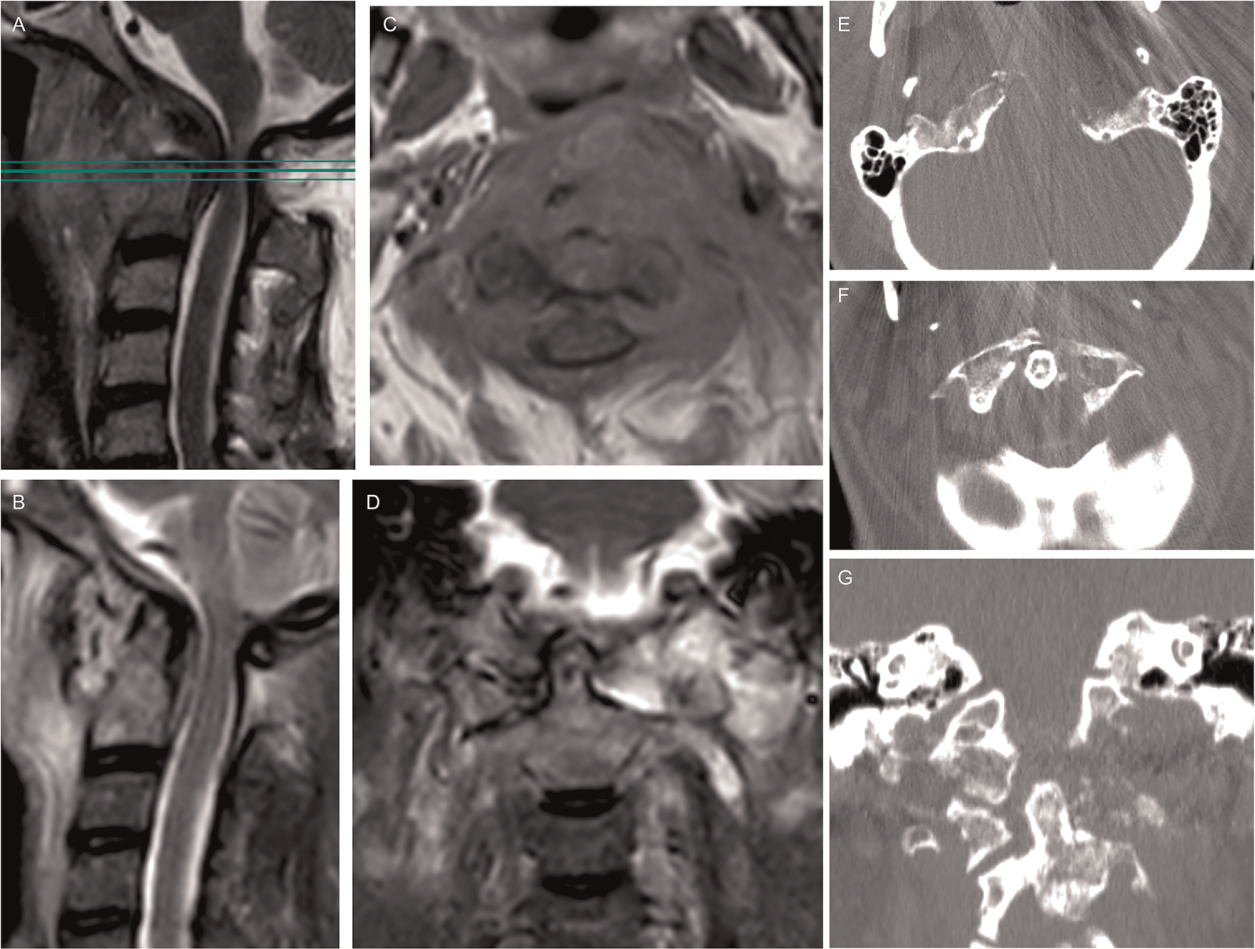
Preoperative spine MRI showed a compression of the midbrain at the craniovertebral junction on the STIR sequence (A); an axial section contrastenhanced T1-weighted (C) depicted a low-enhancing lesion with extension from the clivus, left petroclival fissure, and left condyle up to the prevertebral plane of the fifth cervical vertebral body, determining an anterior dislocation of the oropharynx and an osteolysis of the left part of anterior C1 arch; the lesion showed an hyperintensity in T2-weighted images (B, D). Preoperative CT scan showed an osteolytic involvement of the left condyle and lateral mass (E-G) causing instability of the craniovertebral junction.
